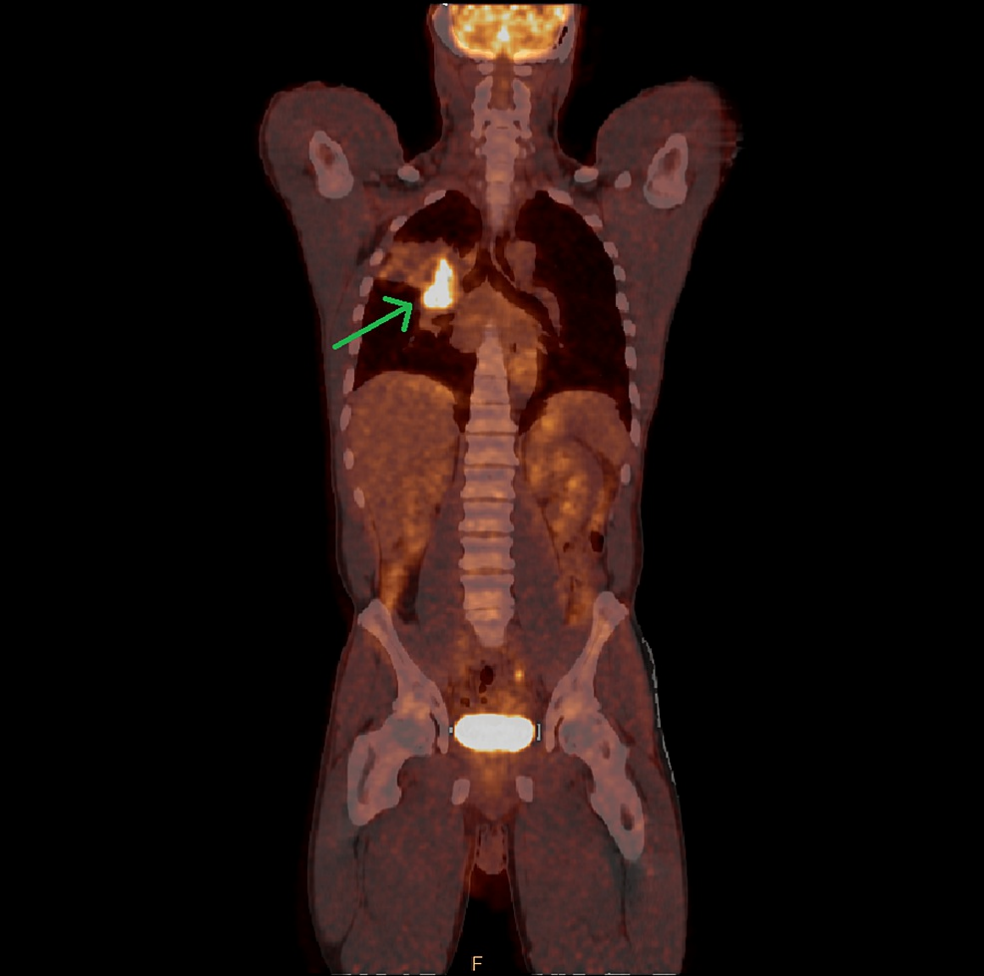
Whole-body 18F-fluorodeoxyglucose positron emission tomography (FDG-PET)/CT demonstrating the FDG-avid right suprahilar mass (green arrow) with a maximum standardized uptake value (SUVmax) of 22.1
